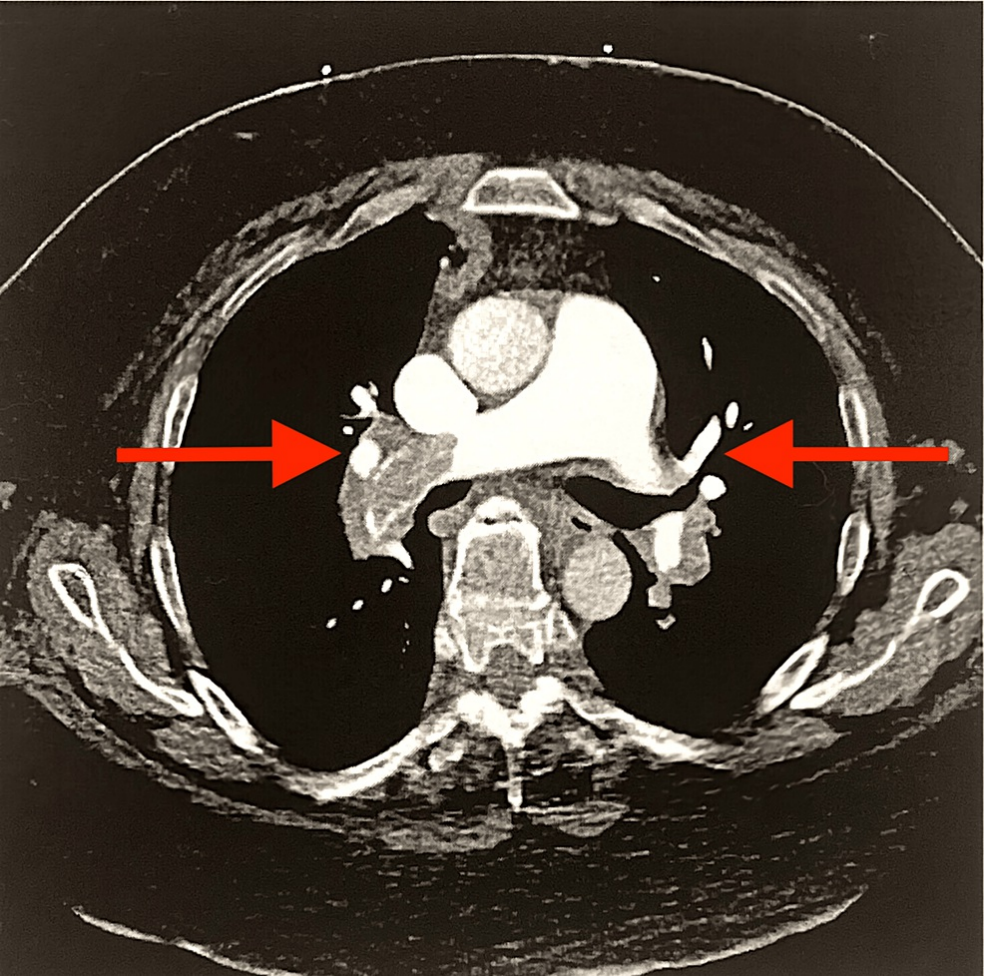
CT pulmonary angiogram showing PE PE, pulmonary embolism
